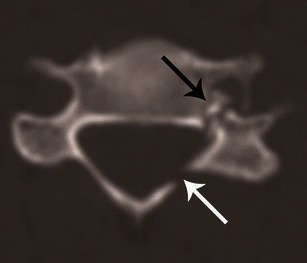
Pediculolaminar separation. Axial CT image shows simultaneous fracture of left lamina (*white arrow*) and pedicle (*black arrow*) of the C5 vertebra, resulting in pediculolaminar separation. Extension of the fracture line to the left transverse foramen and presence of fracture fragment within the transverse foramen seen, raising suspicion for the left vertebral artery injury
